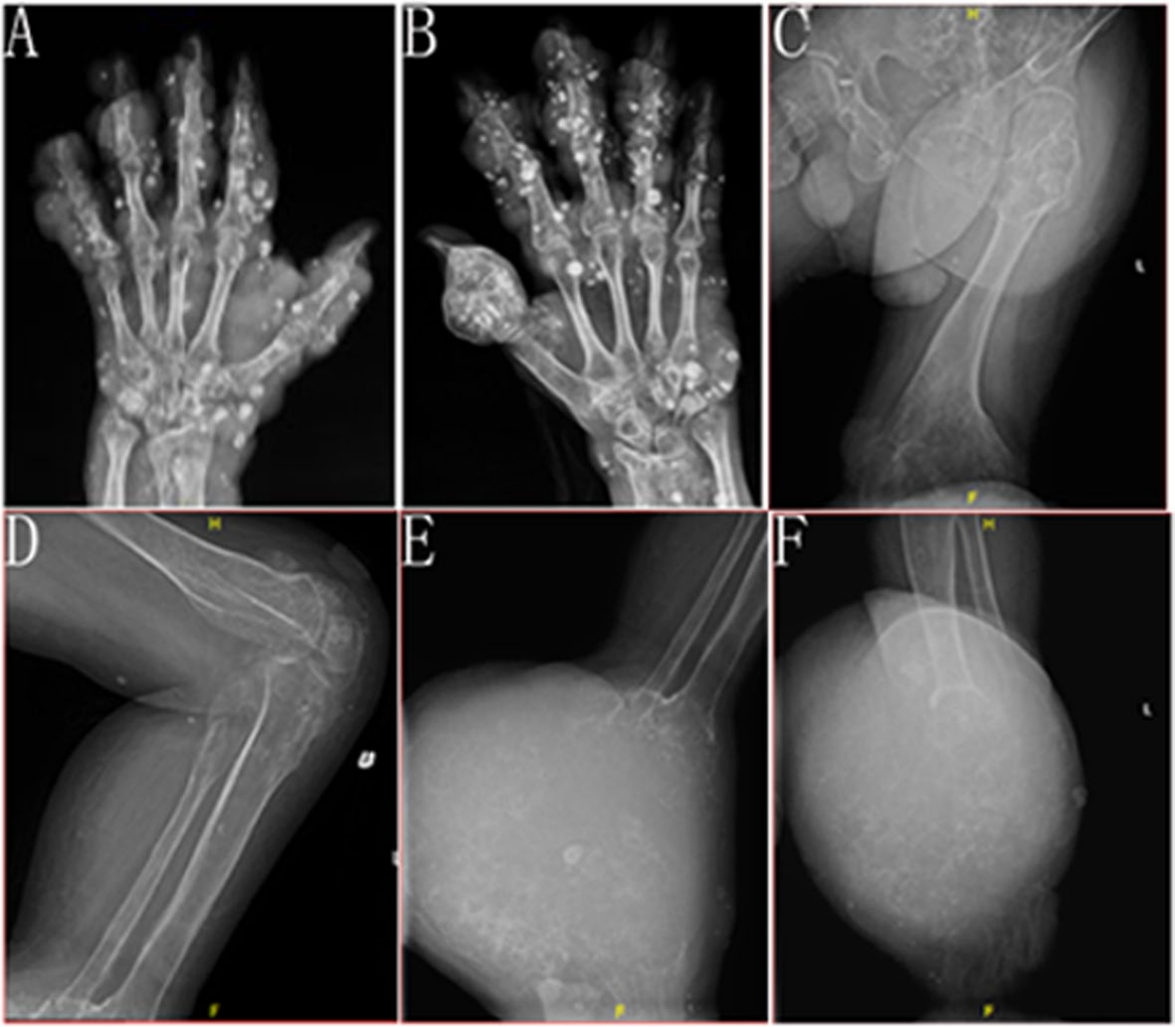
CT examination. A, B Spherical expansive bone destruction in the right thumb and multiple phleboliths in the hands and wrists. C Bone density and morphological changes of the left ilium and left femur. D Multiple venous stones around the left knee joint. E, F Spherical expansive bone destruction with multiple calcifications at the left tarsal
